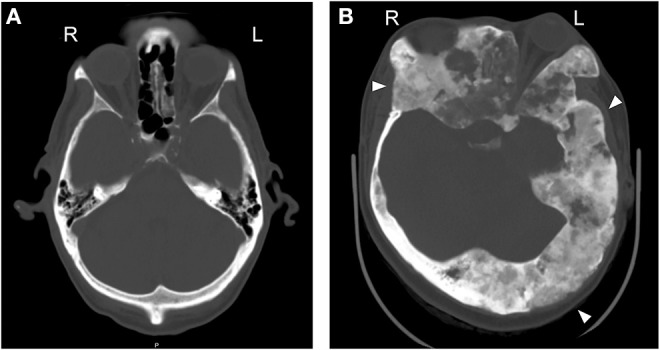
Fibrous dysplasia commonly affects the skull and is mosaic. Axial CT across the orbit. (A) A normal CT scan of the craniofacial bones, from a skeletally normal 61 y.o. male. (B) A 29 y.o. female with craniofacial FD. Note the asymmetry in the skull [ground glass expanded lesions (arrowheads) and displaced left globe].
